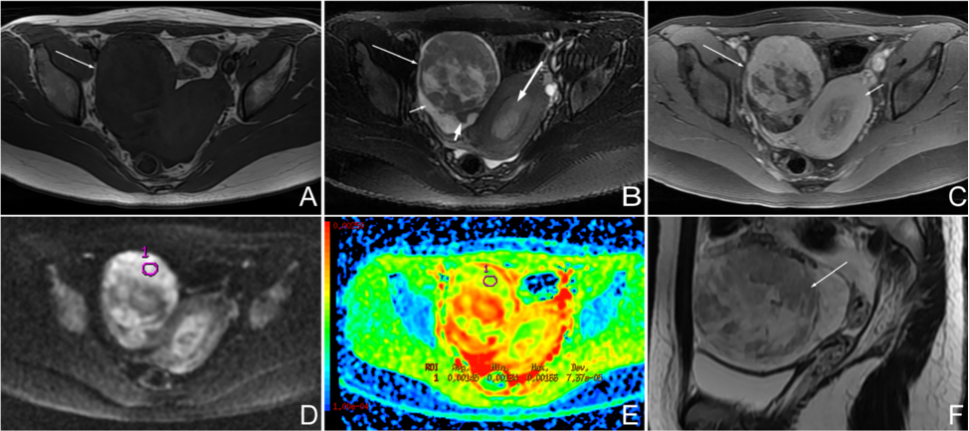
Female, 18 years, luteinized thecoma on the right ovary. a Axial T1WI showed a relatively large mass with isointensity (*arrow*). b On axial fat saturated T2WI, the mass (*long arrow*) showed heterogeneous isointensity compared with the myometrium. Hyperintensity in the mass represented degenerative changes. Minor-volume of ascites (*short arrow*) and ipsilateral ovary (*short bold arrow*) were present. This mass was accompanied with endometrial hyperplasia (*long bold arrow*). c On axial contrast-enhanced fat-saturated T1WI, the mass revealed marked enhancement (*long arrow*), similar with the myometrium (*short arrow*). d On DWI, the mass showed very high signal (grade 3). e On ADC map, the mean ADC value was 1.45 × 10^−3^ mm^2^/s. f On sagittal T2WI, the manifestation was similar to Fig. 3b
